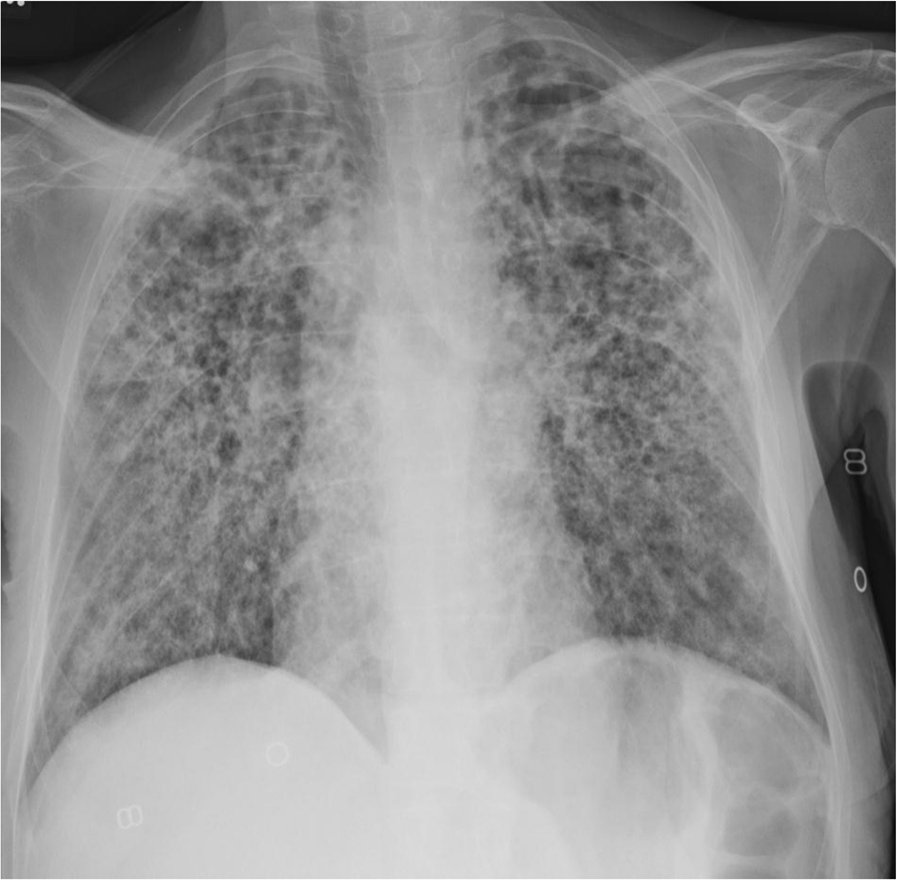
Anteroposterior chest radiograph taken at time of admission. This image shows diffuse bilateral interstitial and airspace opacities.
